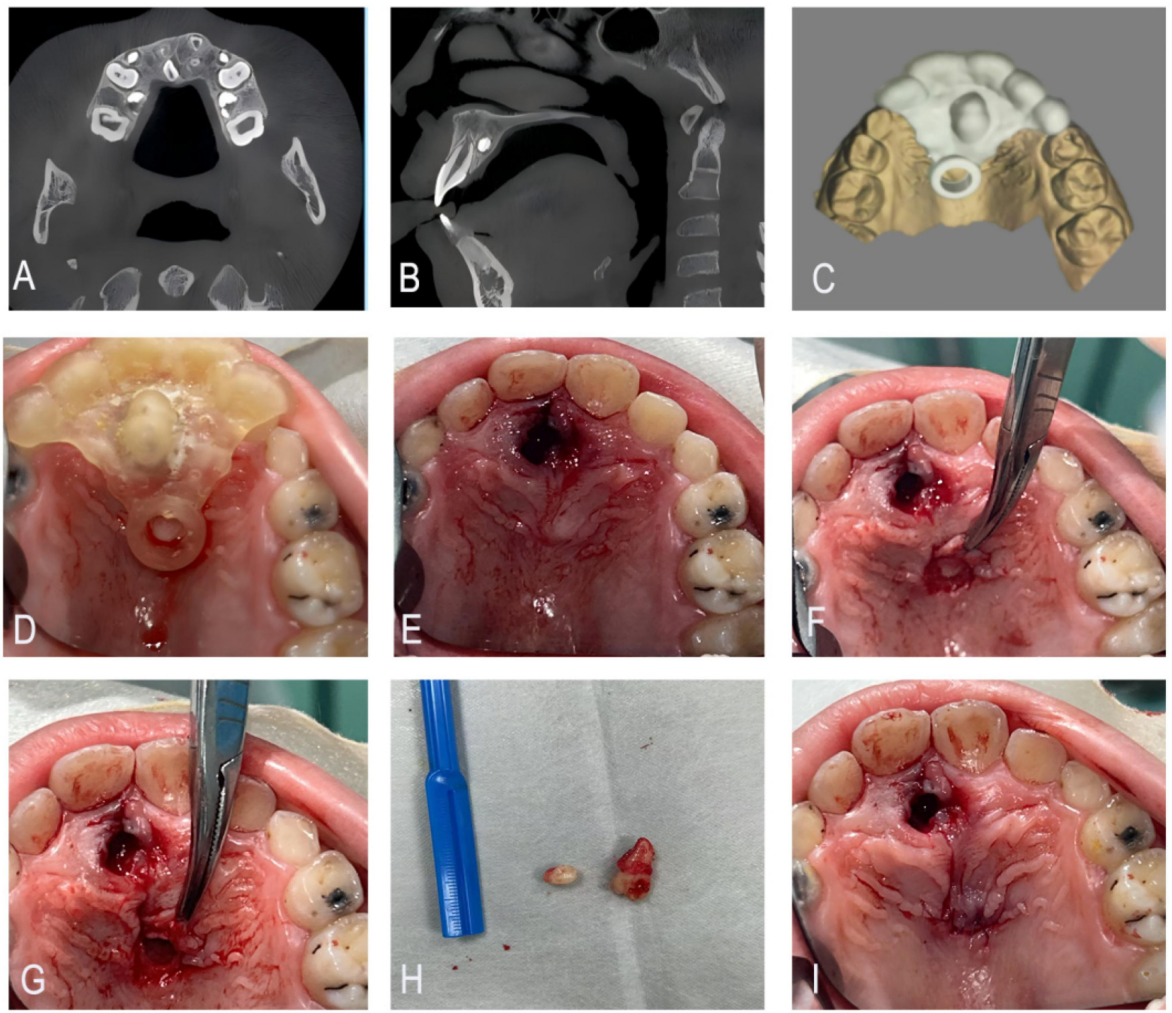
(A,B) CBCT showing a fully developed supernumerary tooth on the palatal side of tooth #21 and a spherical supernumerary tooth apical to the root of tooth #11. (C) Guide plate designed by 3D printing. (D–F) Place the 3D guide plate, flip the flap along the indicator ring and remove the bone covering the surface of the superimposed tooth. (G–I) Extract the supernumerary teeth and suture the incision.
